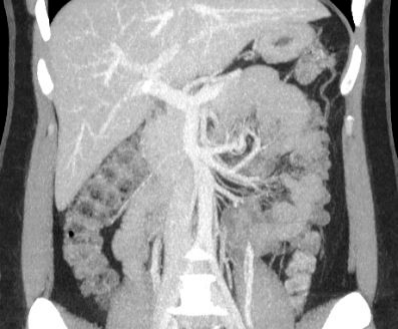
Abdominal computed tomography image in the portal phase, coronal plane, showing the right anterior branch of the portal vein originating from the left branch.
